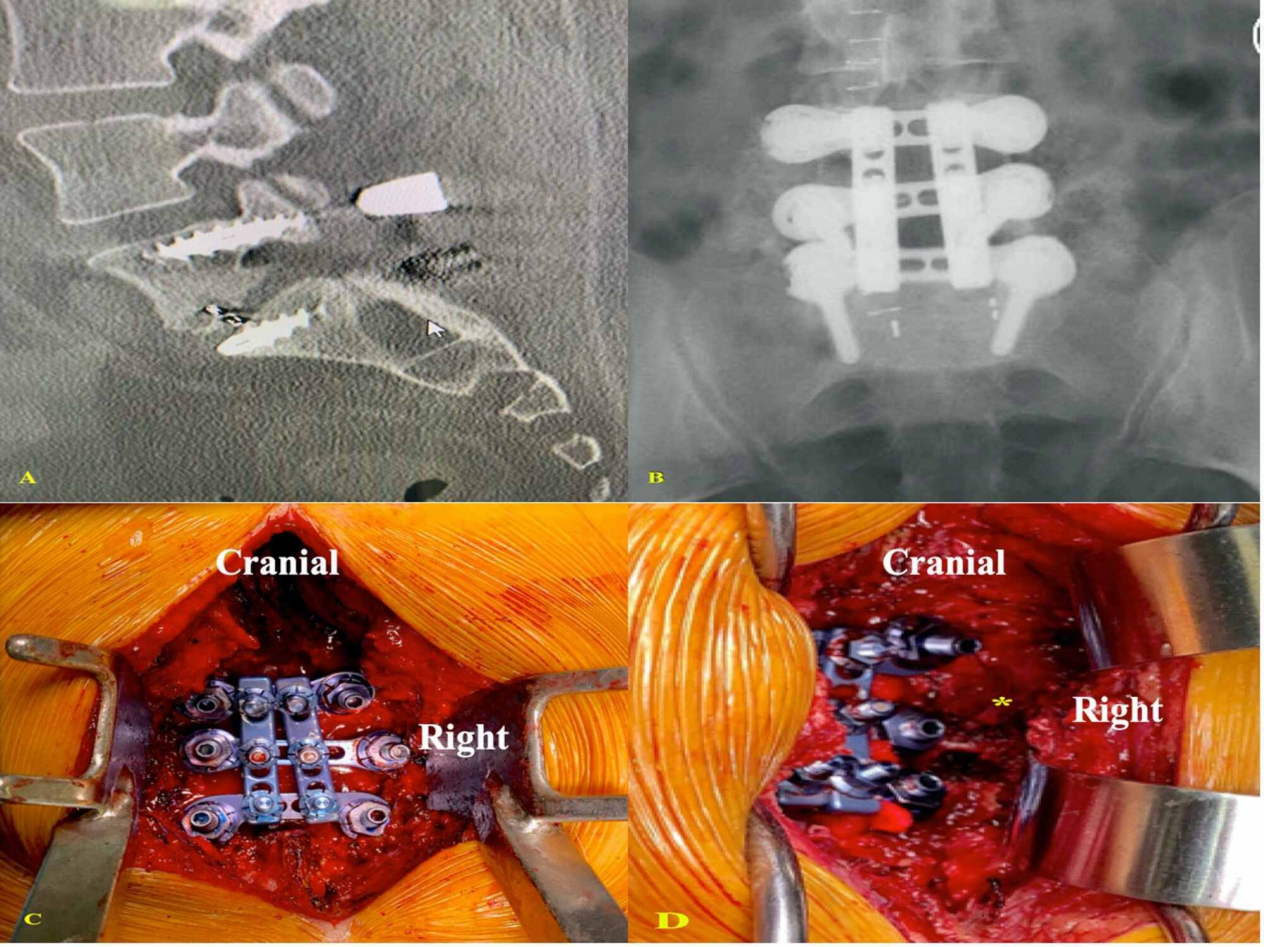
(A) CT lumbar spine (sagittal view) shows evidence of construct failure. (B) X-ray (AP view) shows the UNIMAX system after revision. (C) Intraoperative picture shows the UNIMAX system after placement. (D) Intraoperative picture shows the instrumentation-free large bony surface area (*) in the lateral region, which promotes fusion AP: anteroposterior
